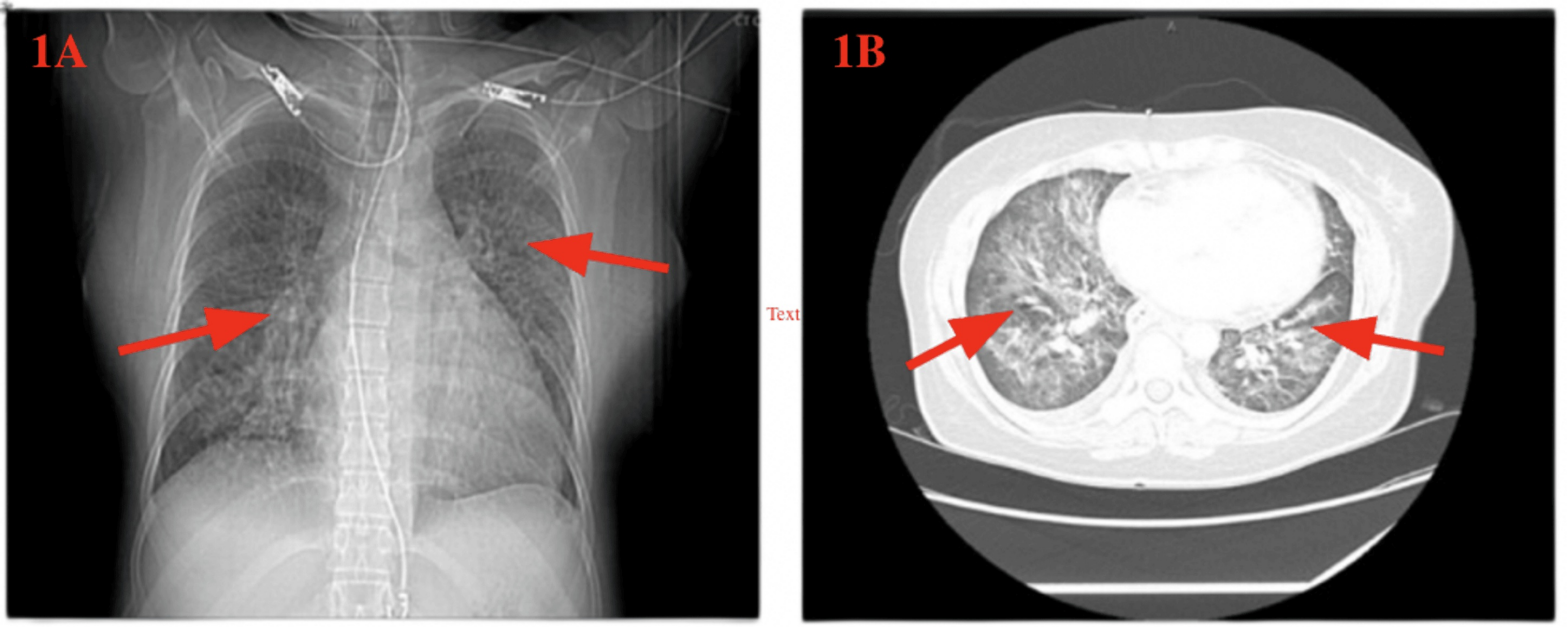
Lung imaging findings in Pneumocystis jirovecii pneumonia (A) Chest X-ray demonstrating hazy, patchy bilateral pulmonary opacities. (B) Chest CT scan demonstrating widespread ground-glass opacities in both lungs. These findings are suggestive of *Pneumocystis jirovecii* pneumonia in a patient with a very low CD4 count. CT, computed tomography; CD4, cluster of differentiation 4
